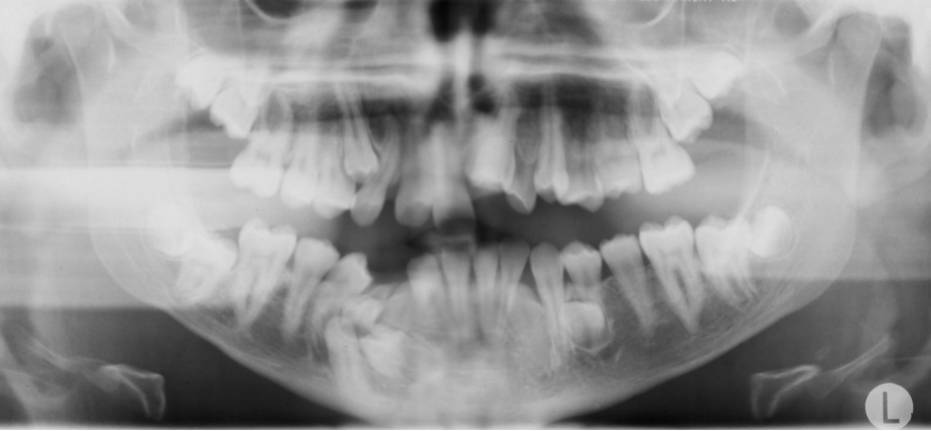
Orthopantomography at 14 years 6 months of age.
